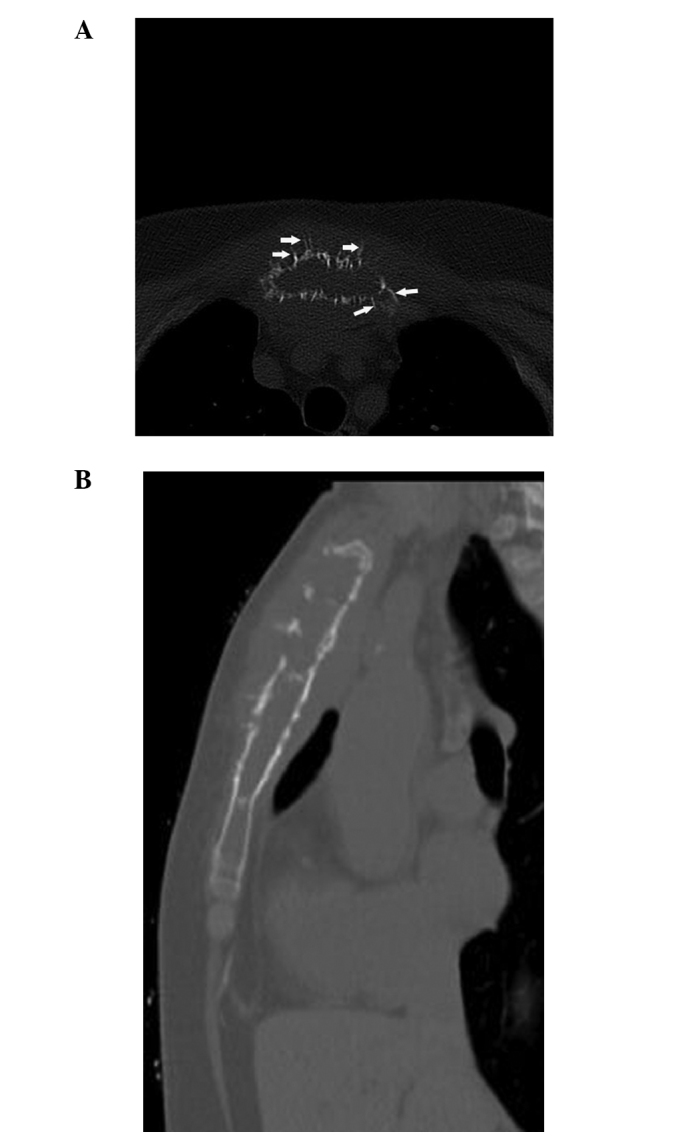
(A) Axial computed tomography (CT) image of the manubrium sterni obtained through the bone window showing an osteolytic lesion with expansion and a periosteal reaction with the appearance of sunrays around the periphery (arrows). (B) Sagittal reconstructed CT image of the sternum showing the lesion involving the manubrium and almost all the body of the sternum. The lesion is slightly expansile and the cortex is partially destroyed.
